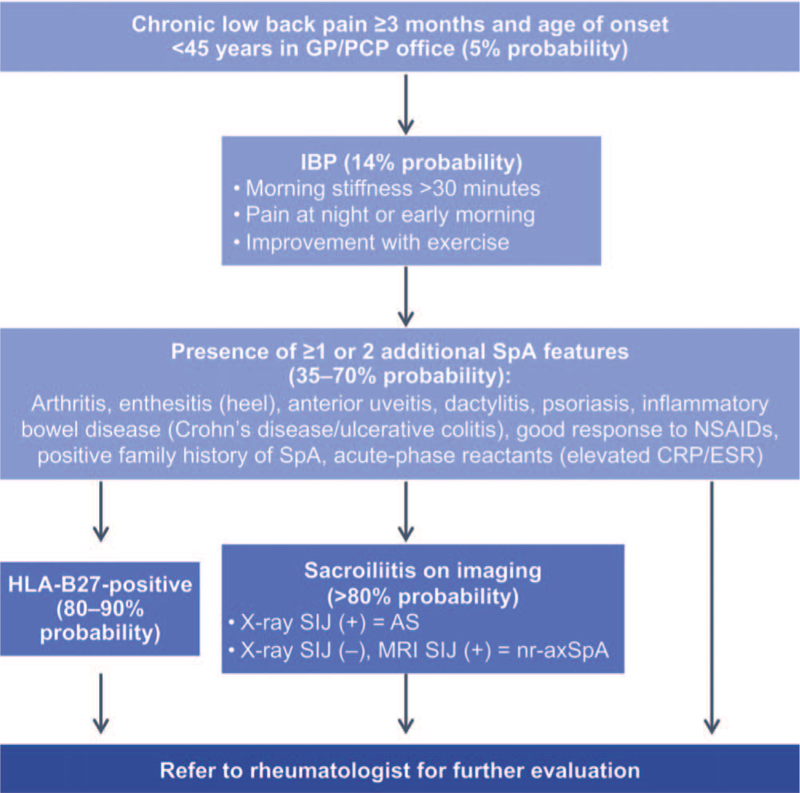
Referral strategy and clinical diagnosis of axSpA. The diagnosis of axSpA can be challenging and is highly dependent on clinical experience and intuition of the treating physician.^[38]^ The first step involves evaluating a patient with chronic back pain to determine whether the back pain is inflammatory or mechanical in nature.^[38]^ IBP is characterized by morning stiffness for >30 min, pain at night or early morning, and improves with exercise but does not improve with rest.^[2,12]^ For those with IBP, clinicians next evaluate the patient for the presence of SpA features. HLA-B27 testing may be performed, because a positive HLA-B27 test result is associated with an increased likelihood that the patient has axSpA.^[2]^ Imaging is also important for the recognition and diagnosis of axSpA. Patients with AS will have structural damage of the SIJ that is evident on X-ray. Patients with nr-axSpA will not have sacroiliitis evident on X-ray, but may have evidence of sacroiliitis by MRI.^[2,3]^ For those with chronic back pain for at least 3 months, an age of onset before 45 years and features of SpA, the presence of objective signs of inflammation (such as elevated CRP and evidence of sacroiliitis by MRI) can help improve the confidence of an axSpA diagnosis. This figure was adapted from Rudwaleit et al^[38]^ (2004). AS = ankylosing spondylitis, axSpA = axial spondyloarthritis, CRP = C-reactive protein, ESR = erythrocyte sedimentation rate, GP = general practitioner, HLA-B27 = human leukocyte antigen-B27, IBP = inflammatory back pain, MRI = magnetic resonance imaging, nr-axSpA = nonradiographic axial spondyloarthritis, NSAID = nonsteroidal anti-inflammatory drug, PCP = primary care practitioner, SIJ = sacroiliac joint, SpA = spondyloarthritis.
